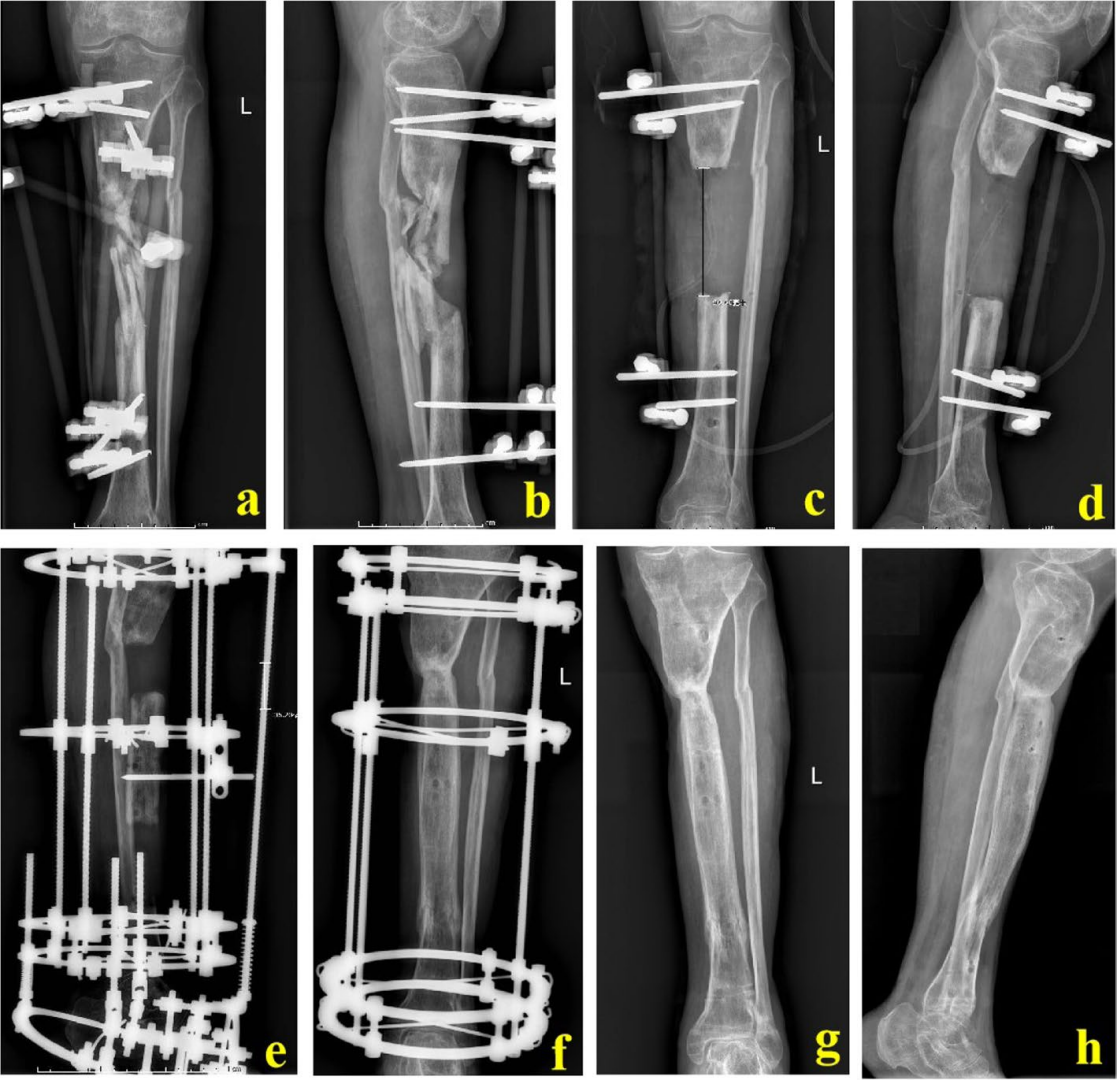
A 53-year-old male was successfully treated by simple bone transport technique. a and b: The patient suffered from a severe open injury and massive sequestrum formed; c and d: After segmental resection of all sequestrum, the bone defect length was 10.5 cm; e and f: Through simple bone transport technique, the bone defects were successfully repaired; g and h: X-ray images after removing the transport frame
